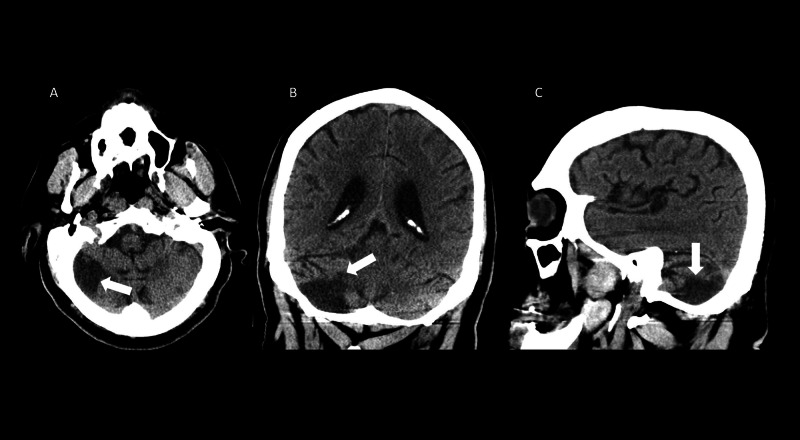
Axial (A), coronal (B), and sagittal (C) computed tomography images demonstrating encephalomalacia in the right cerebellar hemisphere consistent with prior infarct (white arrows).
